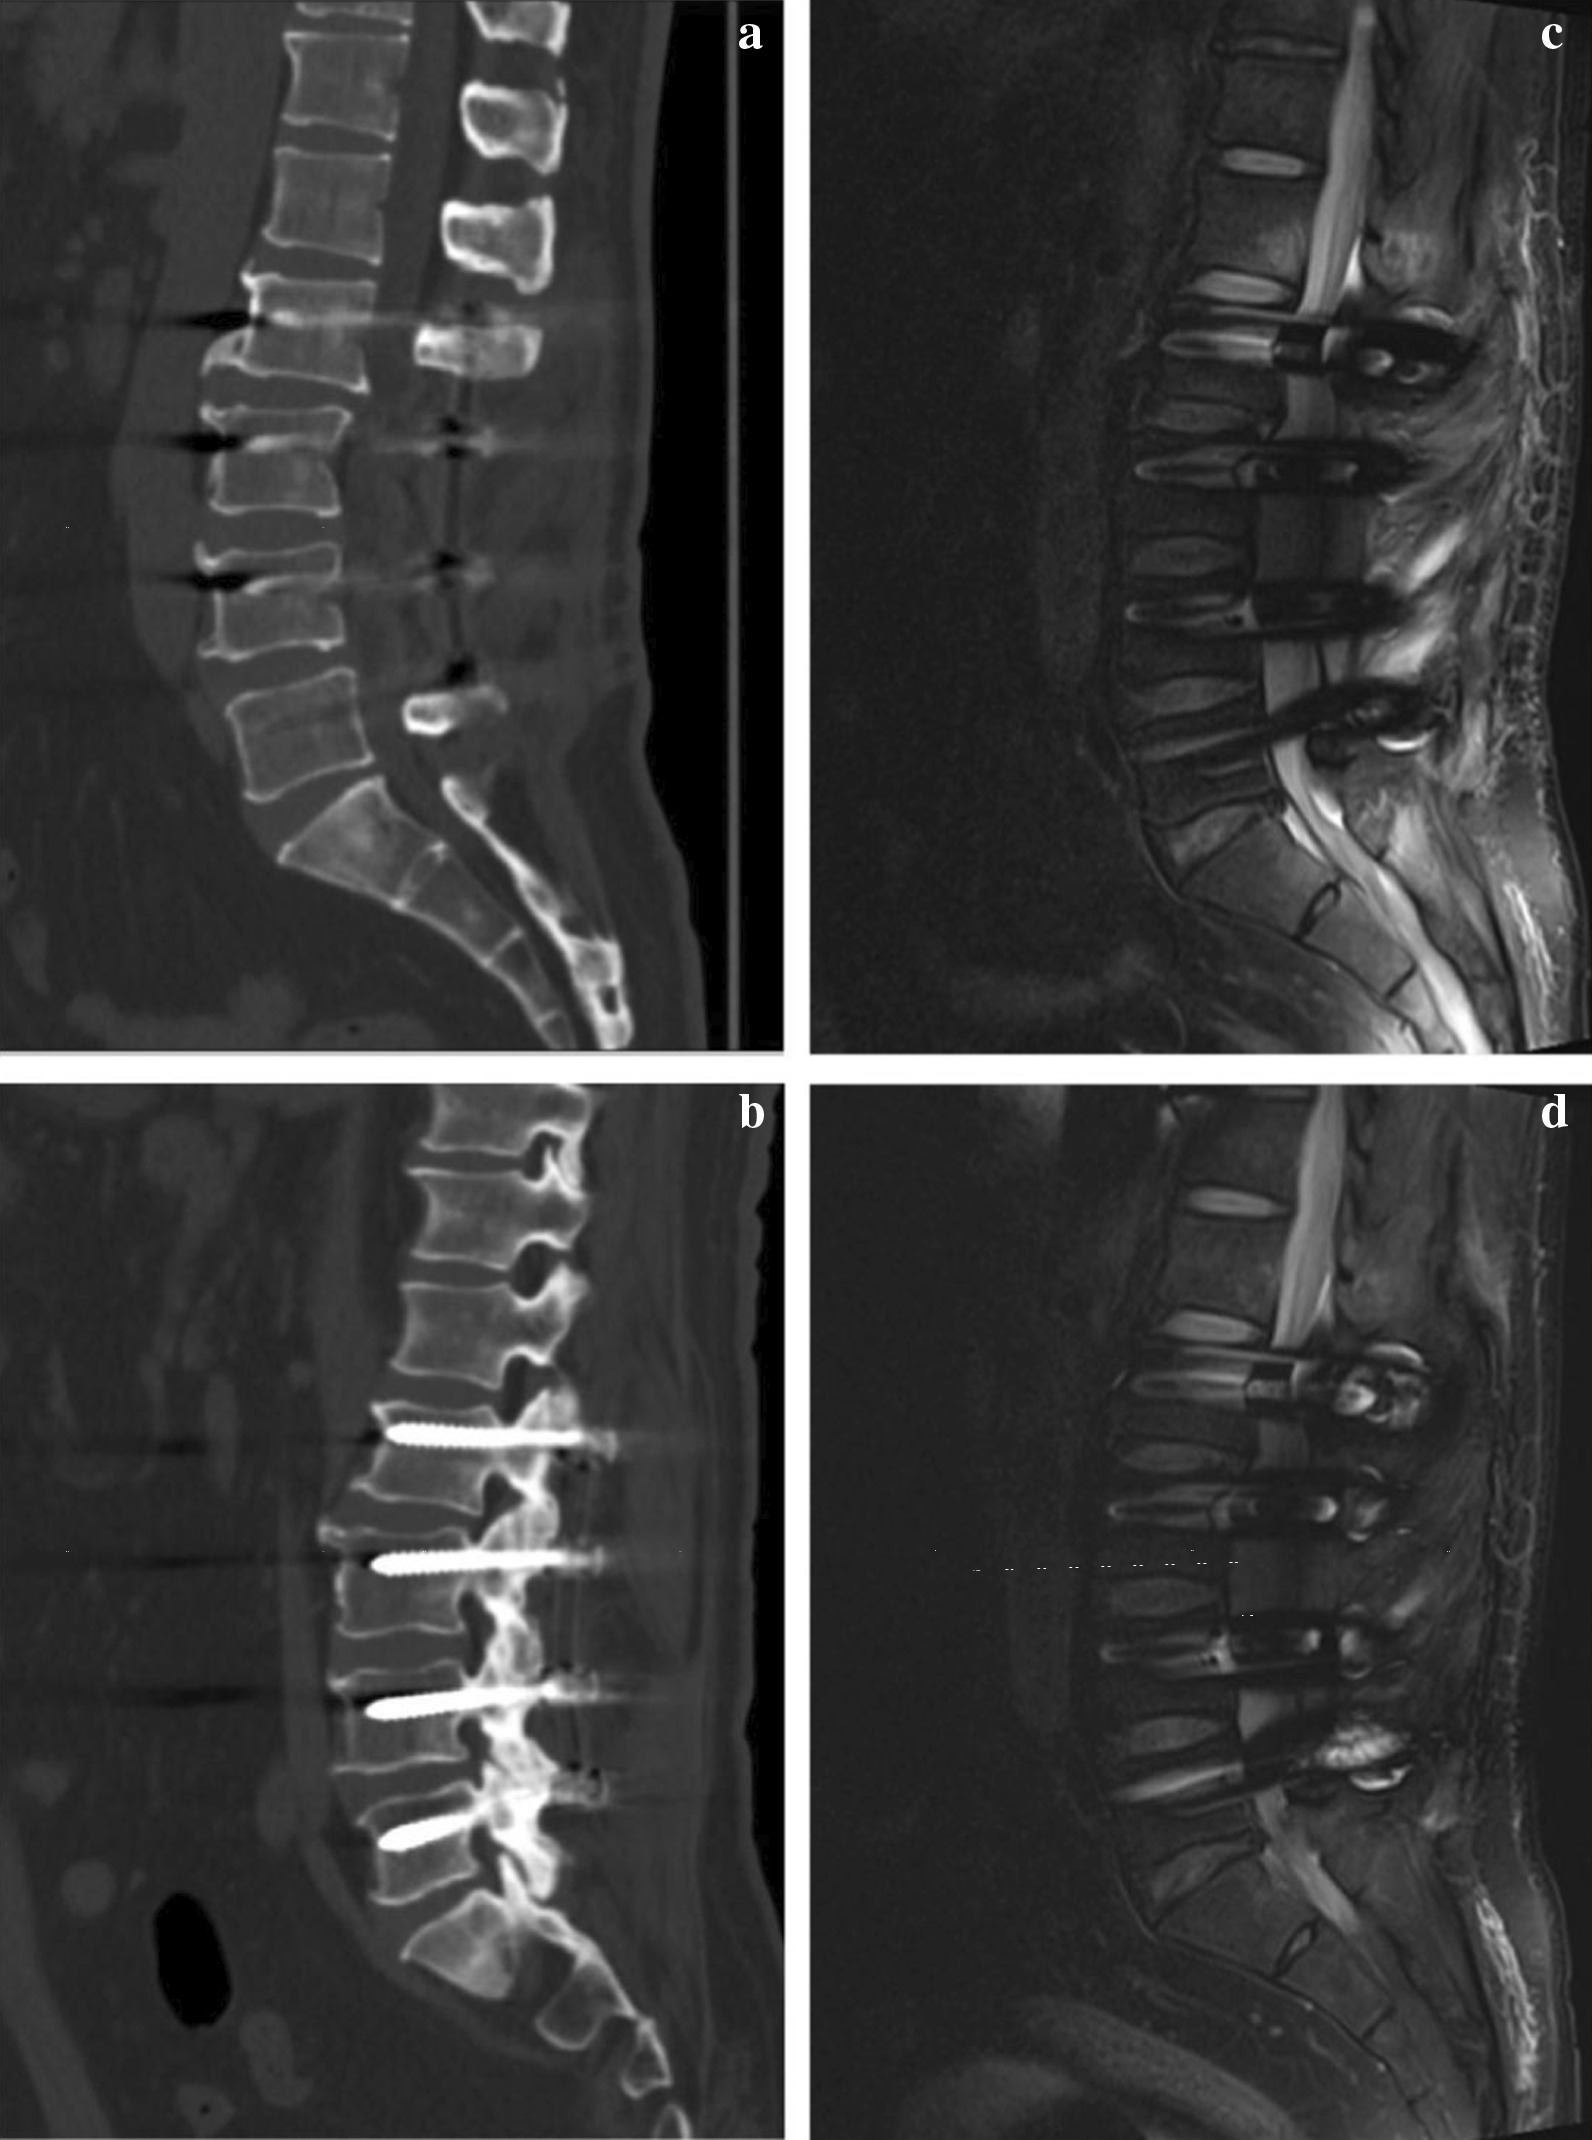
Postoperative CT scan and MRI. Postoperative CT scan shows satisfactory fusion rate of joint facet. MRI has not detected reoccurrence of spinal epidural lipomatosis. a Sagittal CT image of 6 months after surgery. b Sagittal CT image of 22 months after surgery. c Sagittal MRI image of 6 months after surgery. d Sagittal MRI image of 22 months after surgery
